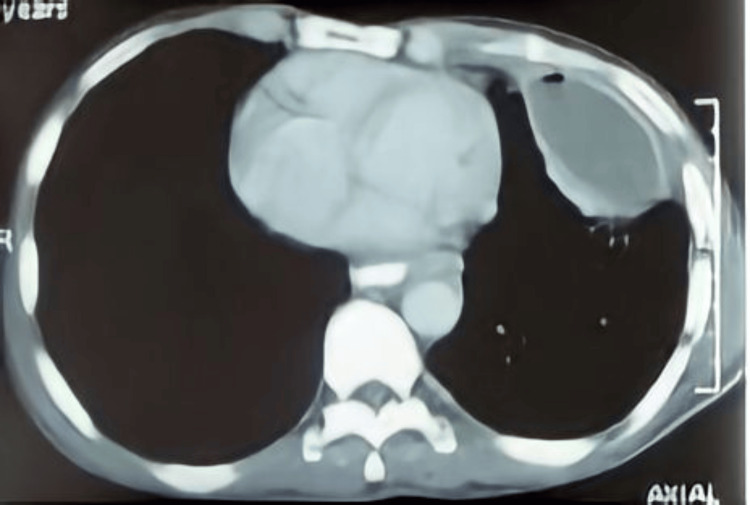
Computed tomography of the chest showing loculated empyema.
